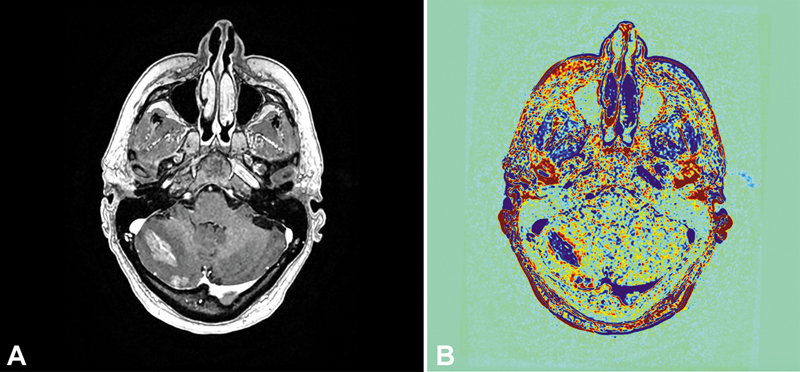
Axial T1-weighted post-contrast image of a cerebellar enhancing lesion (
